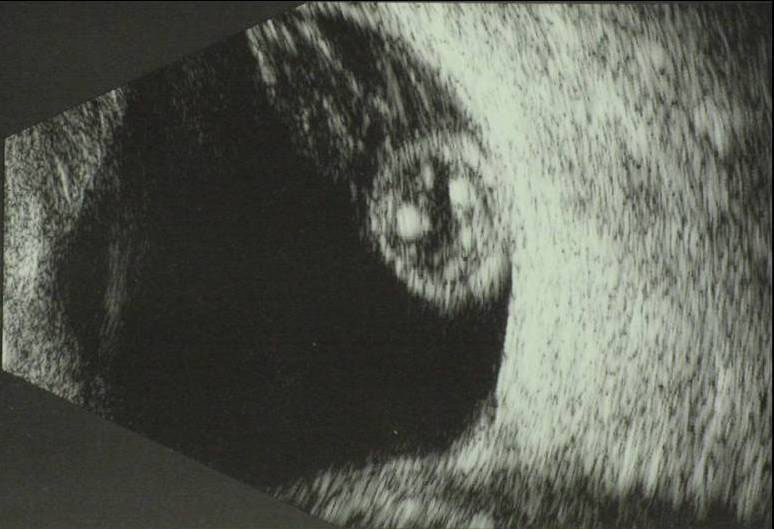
Ocular ultrasound of the left eye demonstrating the dislocated lens in the vitreous cavity. Ocular ultrasound of the left eye at presentation demonstrating the dislocated lens (heterogeneous hyperechogenic oval-shaped mass) within the vitreous cavity (hypoechogenic).
